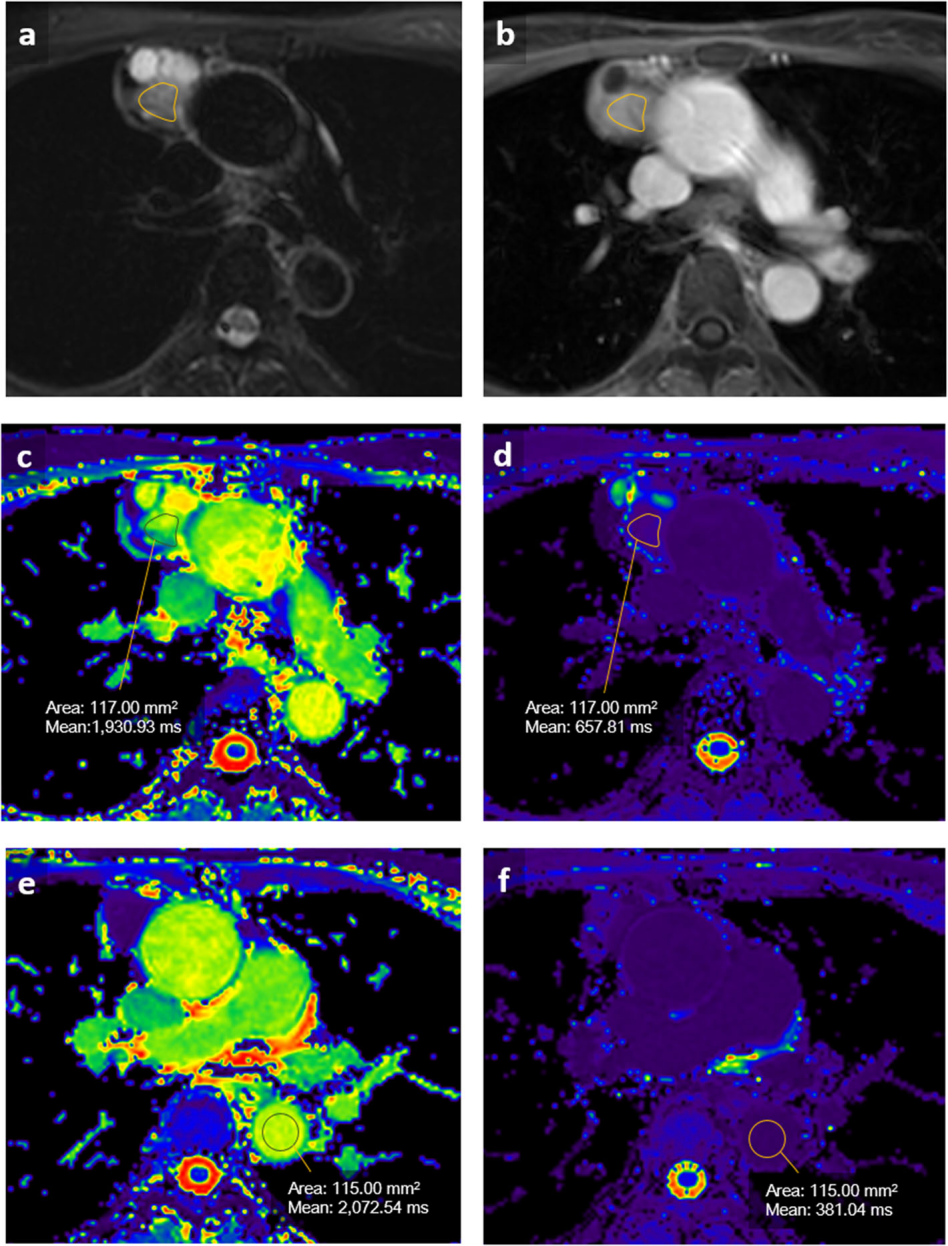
Representative example of a 59-year-old female with type A thyoma. a Axial T2-weighted black-blood imaging. b Axial post-gadolinium T1-weighted images (T1WI). c, e Axial native T1 mapping imaging. d, f Axial post-contrast T1 mapping imaging. The freehand region of interest (ROIs) were manually drawn on both native T1 and post-contrast T1 mapping imaging (c, d) where the largest area of the tumor on axial MR images was included. The ROI was smaller in size than the mass and included only the enhancing part of the tumor avoiding cystic or necrosis part. T1 values of the blood pool were obtained from the descending thoracic aorta at right pulmonary artery level on both native T1 and post-contrast T1 mapping imaging (e, f)
